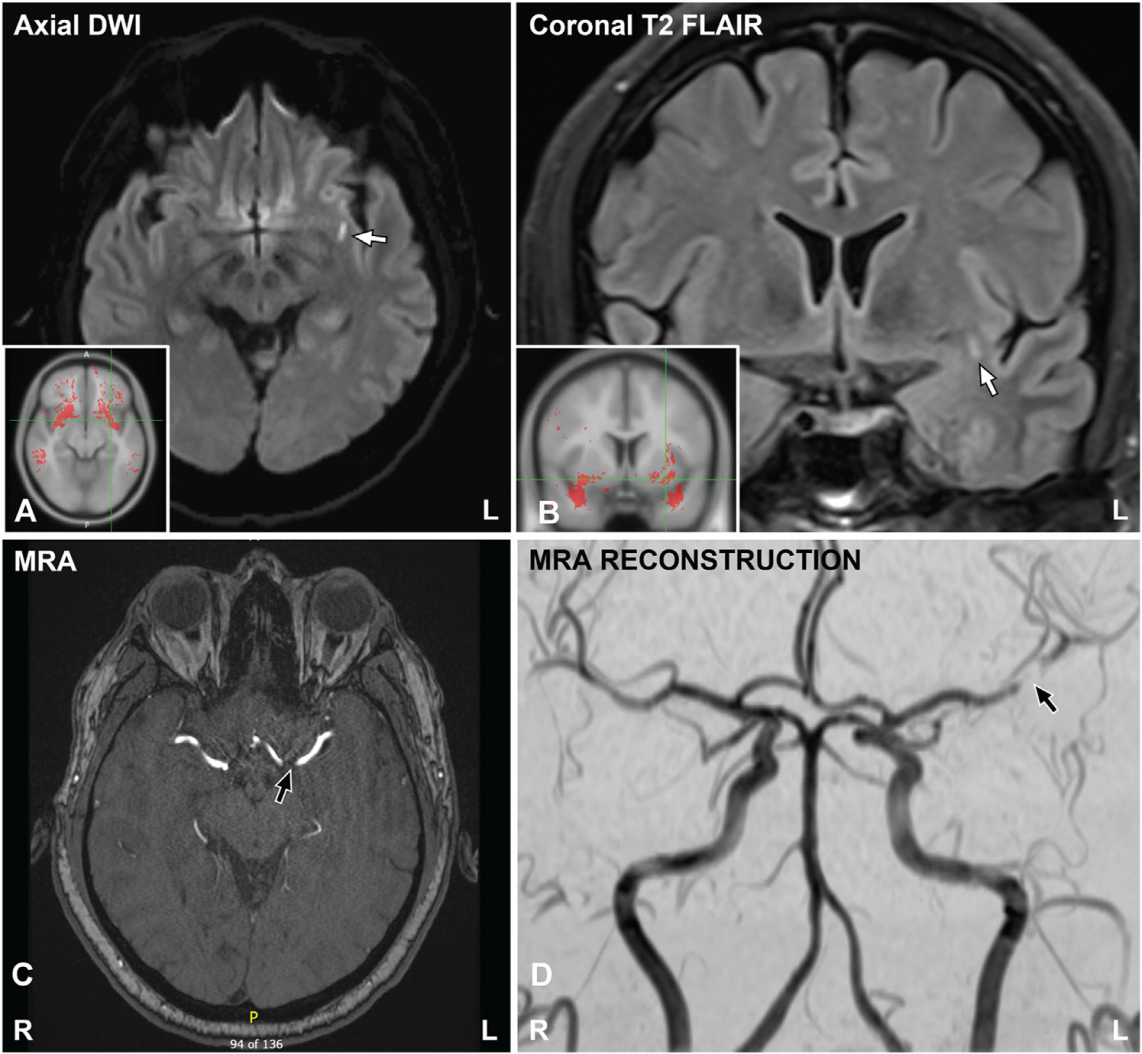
Magnetic resonance imaging and MRA images reveal a subacute left subinsular white matter stroke. A, Axial diffusion-weighted imaging shows T2 bright signal in frontal subinsular white matter, consistent with a recent stroke. B, The presence of a corresponding bright signal on coronal T2 fluid-attenuated inversion recovery image indicates subacute (>6 days) onset. *Inset*: Johns Hopkins University white matter fiber tract atlas show stroke location on a representative white matter atlas, corresponding with the uncinate fasciculus. C, MRA image shows severe stenosis of the middle cerebral artery M2 segment at the origin of the lenticulostriate arteries. D, Vessel reconstruction shows filling distal to the area of blockage, suggesting good collateral flow around an occlusion. *FLAIR*, Fluid attenuated inversion recovery; *MRA*, magnetic resonance angiography.
